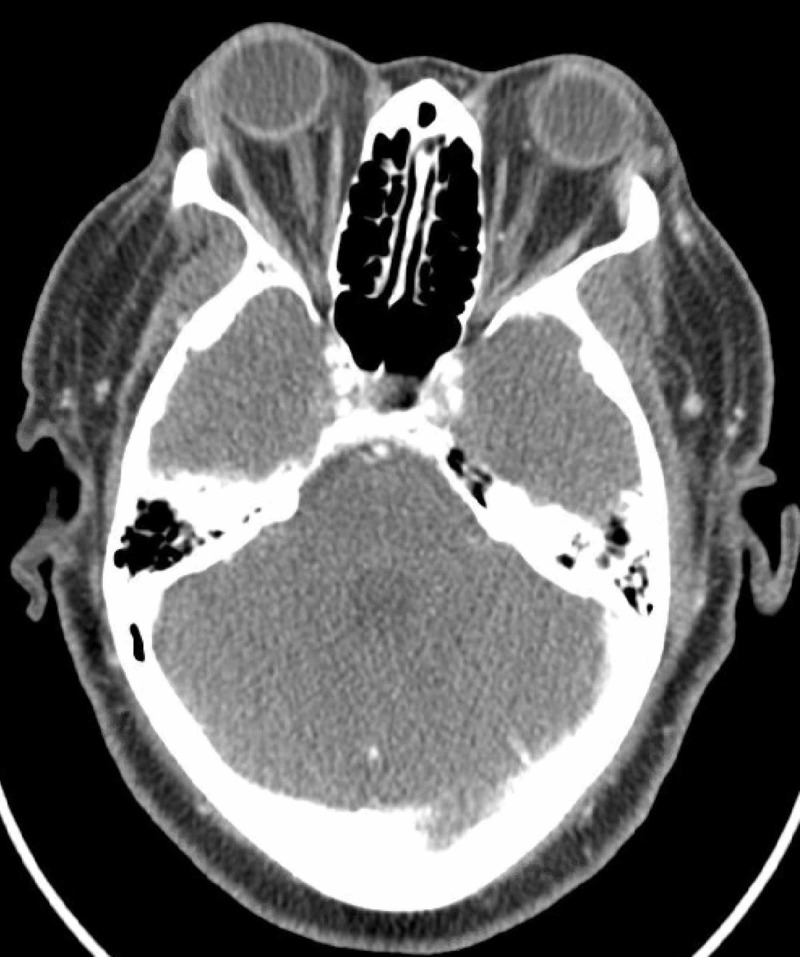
CT imaging of the orbit showing no residual or loco-regional extension of the lesion.
